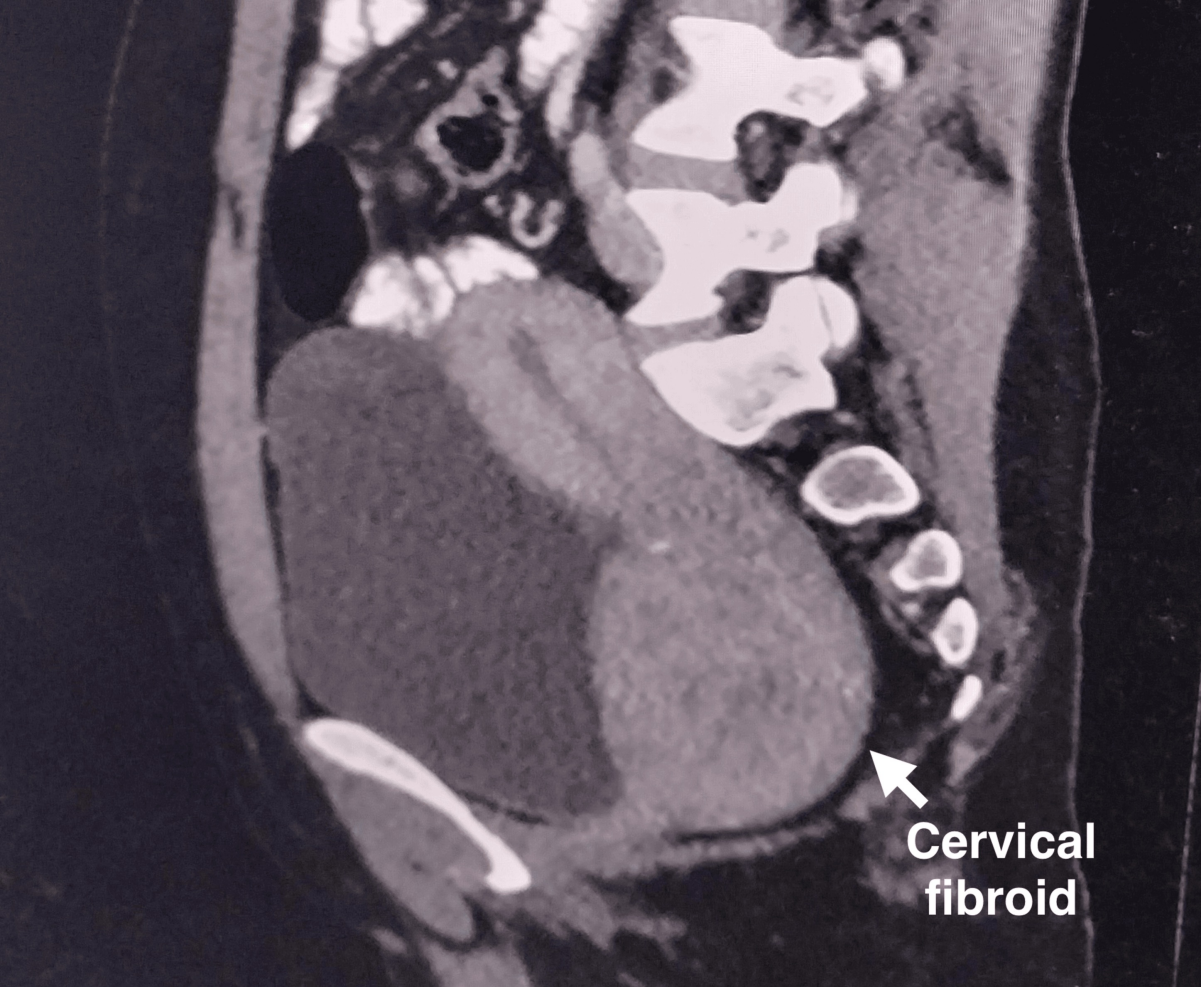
Contrast-enhanced CT revealed a heterogeneously enhancing, whorled appearing, iso to hypo-dense lesion measuring 5 x 6.3 x 6.3 cm in the endocervical region, likely representing a cervical fibroid replacing the anterior lip of cervix
